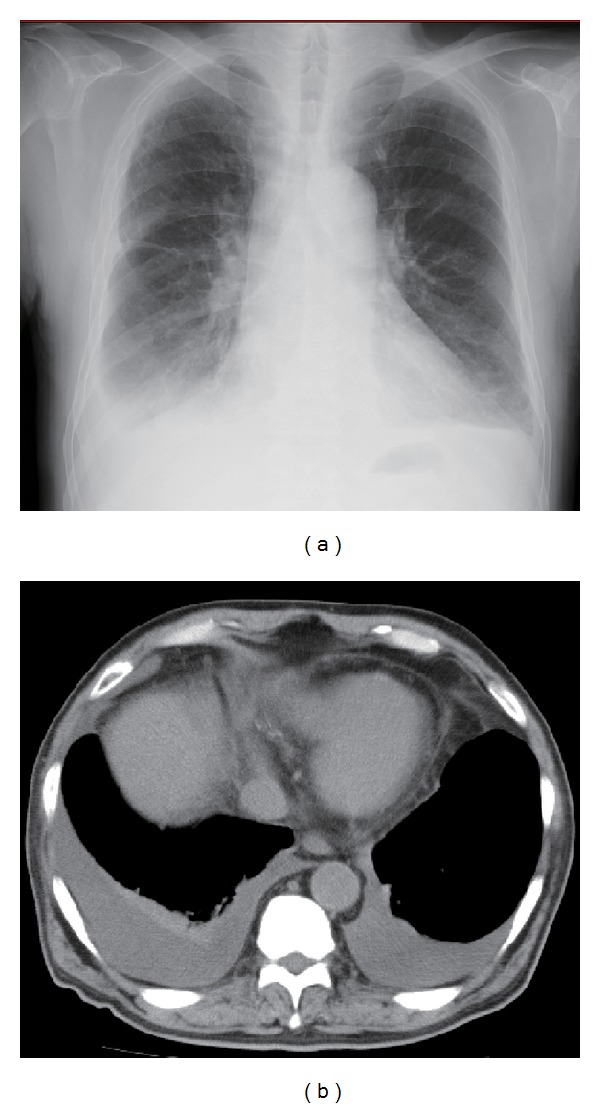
Chest X-ray (a) and computed tomography (b) showed bilateral pleural effusion without any consolidation or ground glass opacities in the lung.
